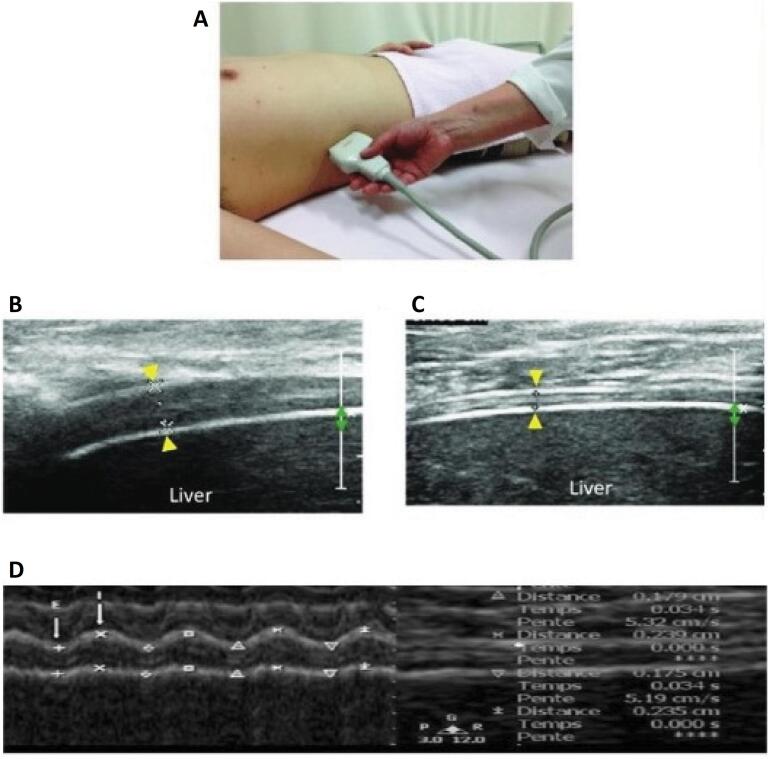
(A) Ultrasound probe position for assessment of diaphragm thickness fraction (DTF); a high-frequency linear probe (7 - 12 MHz) is placed perpendicular to the chest wall at the zone of apposition which corresponds to mid-axillary line, 8th - 11th intercostal space (B) and (C) bidimensional image of the diaphragm during inspiration and expiration respectively; (D) M-mode applied on diaphragm. E - represents the thickness of the diaphragm during expiration. I - represents the thickness of the diaphragm during inspiration.
